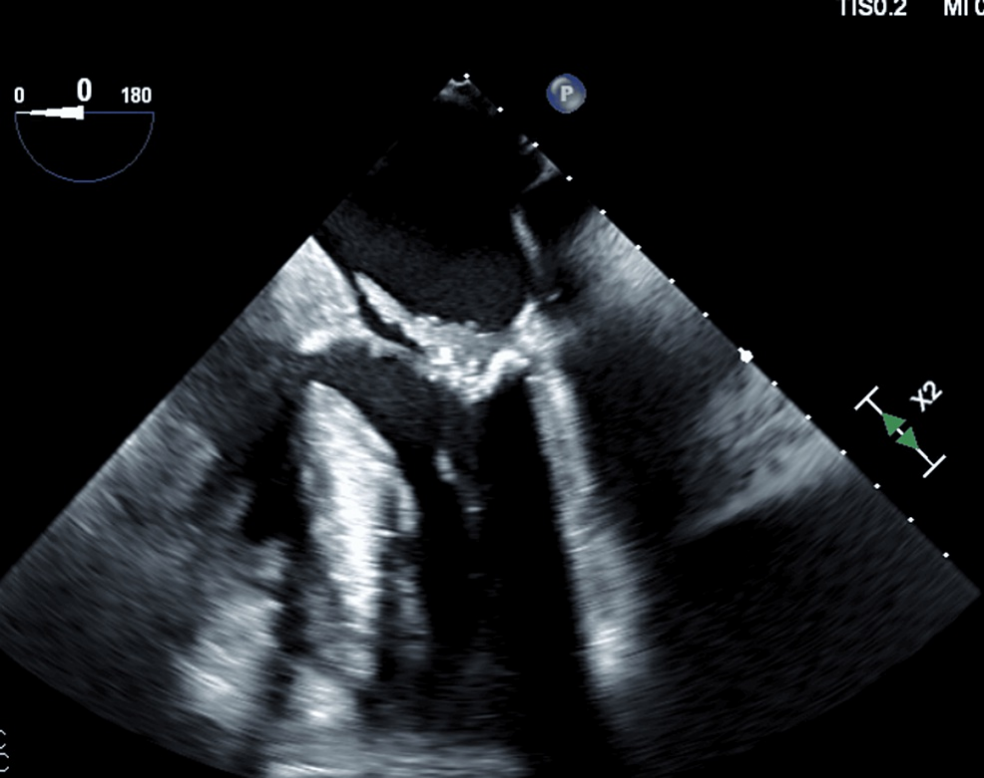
Transesophageal echocardiogram pre procedure showing a large vegetation on the posterior mitral valve leaflet
